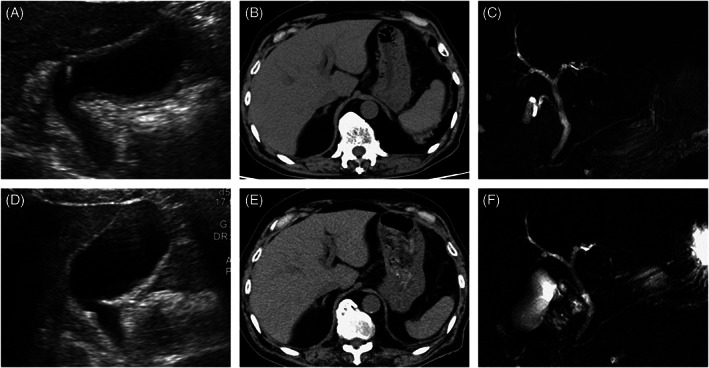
Radiological findings of Case 1: (A–C) are figures at the onset of liver injury: (A) ultrasonography shows diffuse thickening of the gallbladder wall; (B, C) no abnormal findings are present in CT and MRI; (D–F) are figures at the relapse of liver injury; no abnormalities are present in ultrasonography, CT, and MRI
